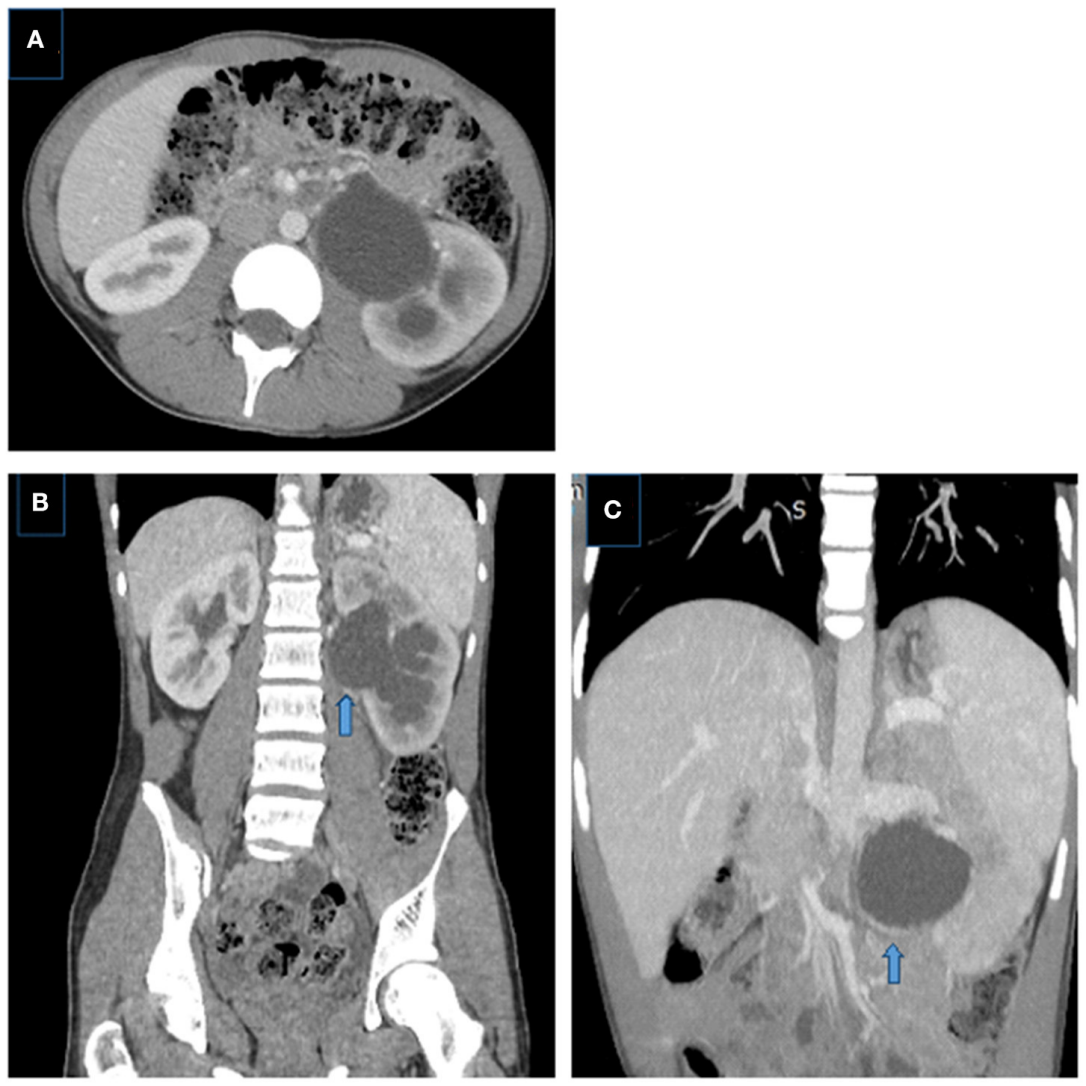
Crossing aberrant renal artery causing left UPJ obstruction in a 14 year-old boy. (A) Axial and (B) coronal CT images showing left pelvicalyceal system dilatation with delayed nephrogram phase, pelvis is extrarenally located, dilatation is more prominent in the pelvis than calices, note the crossing vessel (arrow). (C) Coronal MIP image better demonstrates the crossing vessel as the cause of UPJ obstruction (aberrant lower pole artery) (arrows).
